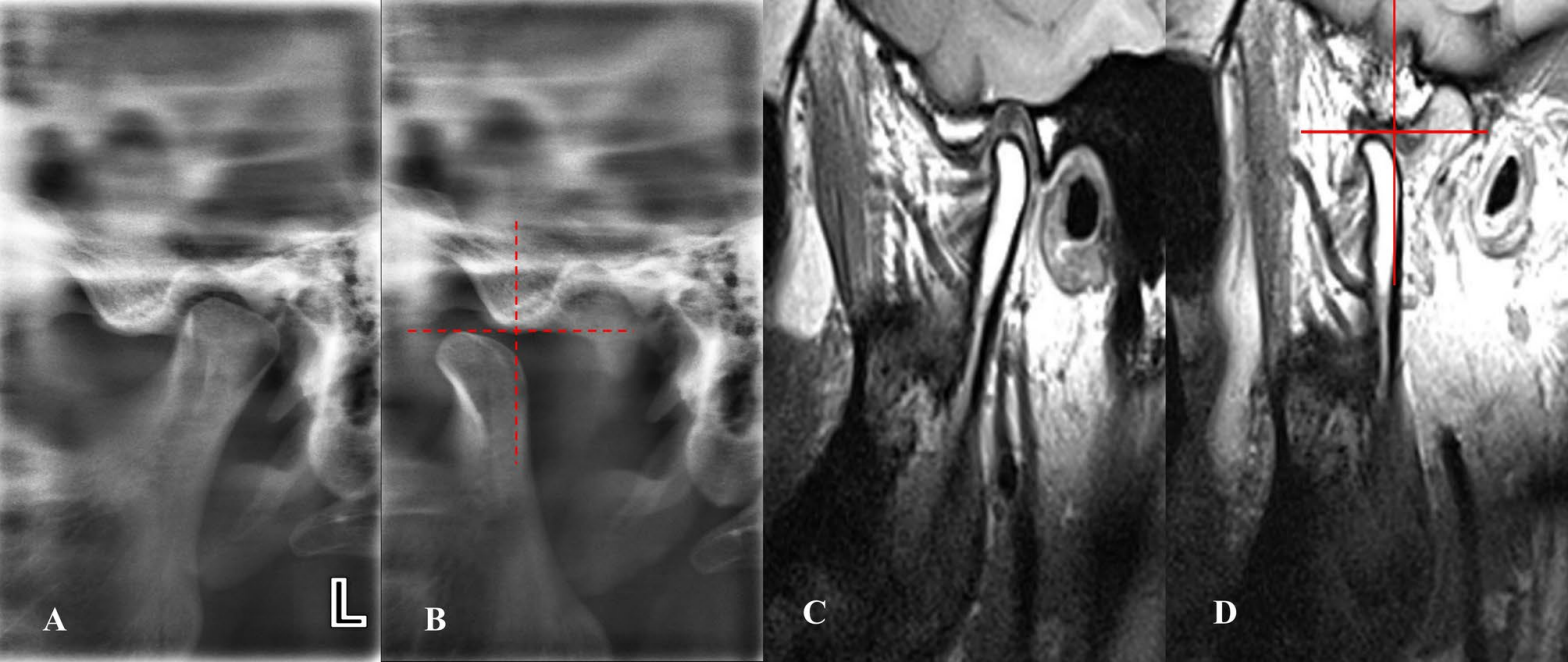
Lateral TMJ view representing Stage I-A. Closed mouth (A) and mouth (B) position show the condyle located anterior to the eminence but at the same level as the highest point of the eminence. Oblique sagittal T1-weighted images representing Stage I-A in closed mouth (C) and open mouth (D) positions show a normal condyle-disk-fossa relationship. The disk-condyle complex is located anterior to the eminence but at the same level as the highest point of the eminence
